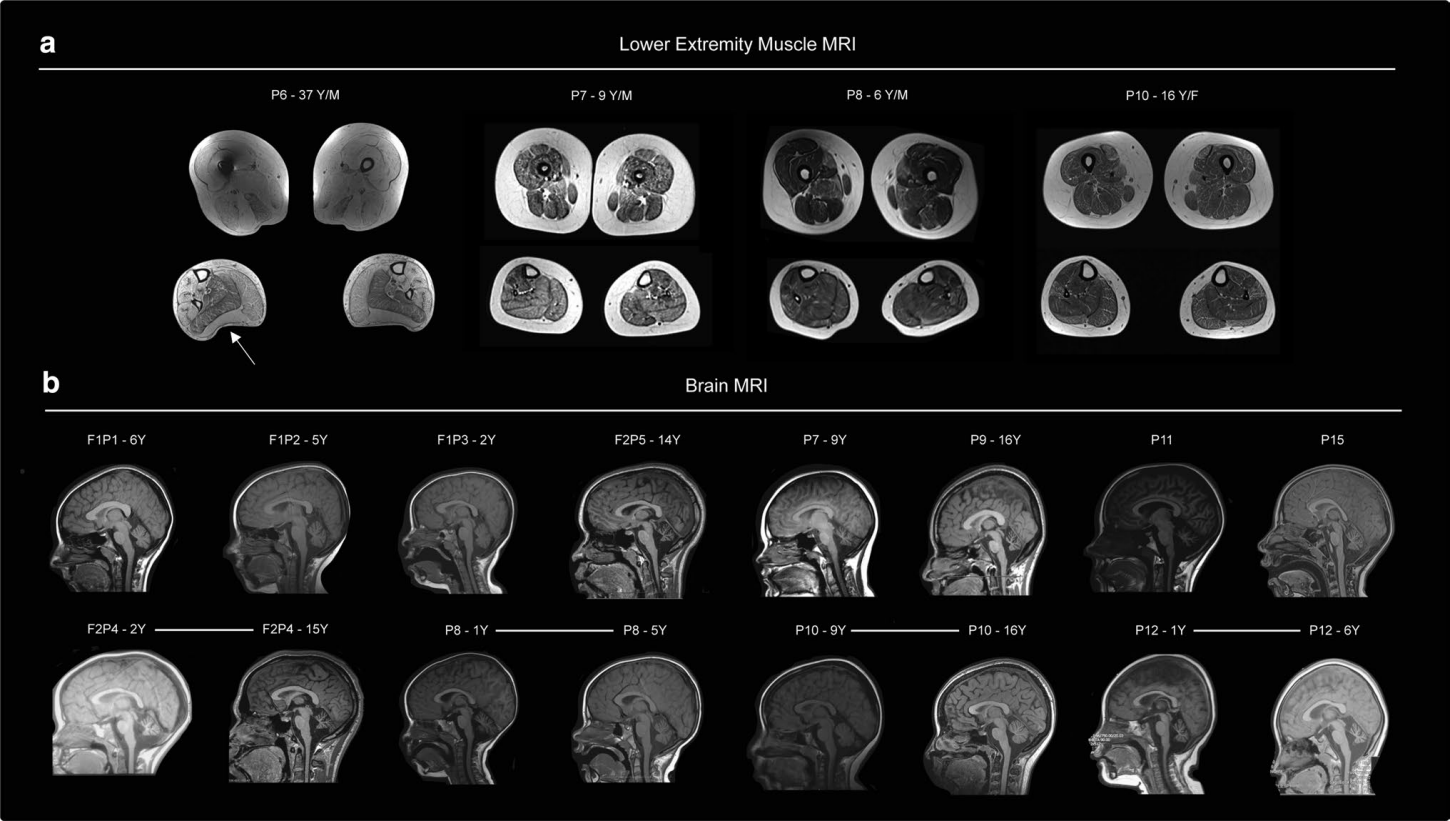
Muscle and brain imaging. a Lower extremity muscle MRI of patients P6 [p.(Asp236His); p.(Phe217Leu)], P7 [p.(Leu450Phe); deletion], P8 [p.(Phe217Leu); p.(Asp236His)] and P10 [p.(Asp236Gly); p.(Arg279His)] at ages 37 years, 9 years, 6 years and 16 years, respectively. Abnormal signal intensity of muscles such as the posterior gastrocnemius muscle in patient P6 (white arrow), reflects muscle breakdown and replacement with adipose tissue. b Brain MRI completed in 12 patients consistently demonstrates moderate-to-severe cerebellar volume loss or hypoplasia involving the vermis and both hemispheres. Repeat MRI images were available in patients P4 [p.(Gly420ValfsX2); p.(Arg256Gln)], P8 [p.(Phe217Leu); p.(Asp236His)], P10 [p.(Asp236Gly); p.(Arg279His)] and P12 [p.(Arg345His); p.(Thr324Ile)] demonstrate mild [P12] to no progression [P4, P8 and P10] of cerebellar volume loss over time (second row)
